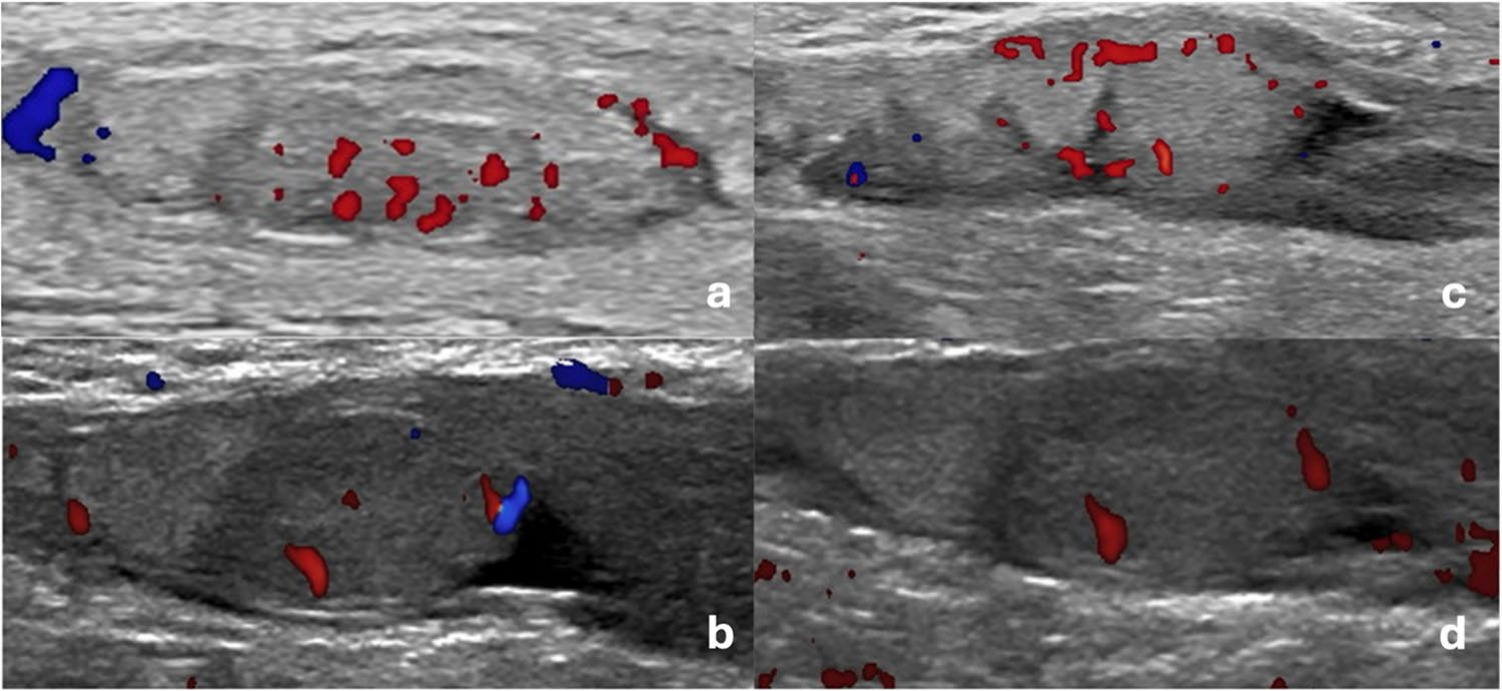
Longitudinal view of a Doppler mode ultrasound of the scrotum performed at day 3 of life, showing adequate vascularity in both testes (a-b left testicle, c-d right testicle)
